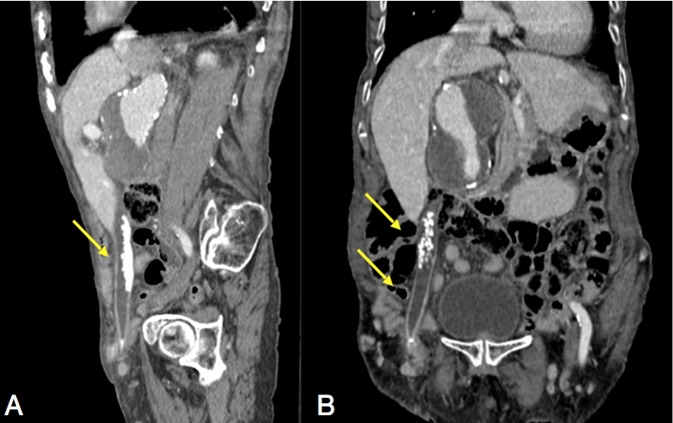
Abdominal CT in the sagittal (A) and coronal (B) axis showing the gallbladder
